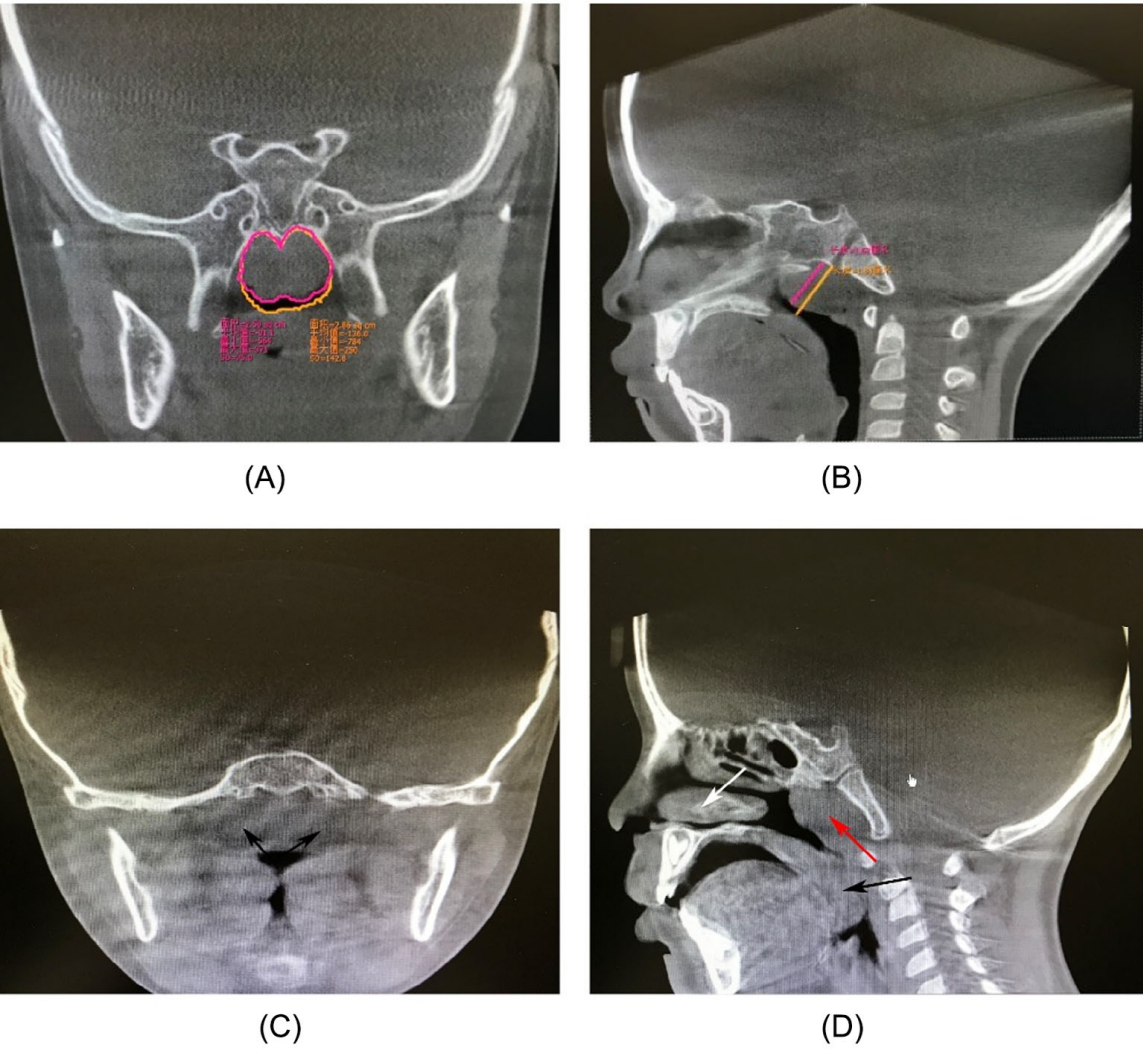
Coronal scan and sagittal reconstruction of CBCT. (A) Coronal scan with hypertrophic adenoids seen at the posterior nostril, the software can automatically calculate the cross‐sectional area (adenoids in the red circle, posterior nostril in the yellow circle). (B) Sagittal reconstruction calculating A/N (red is the thickness of adenoids, yellow is the width of the nasopharyngeal cavity at the most convex part of the adenoids) values. (C) Coronal scan showing hypertrophic tonsils in the oropharynx (black arrows). (D) Sagittal reconstruction showing hypertrophic inferior turbinates (white arrows), hypertrophied adenoids (red arrows), hypertrophied tonsils (black arrows), and airway compression and obstruction.
